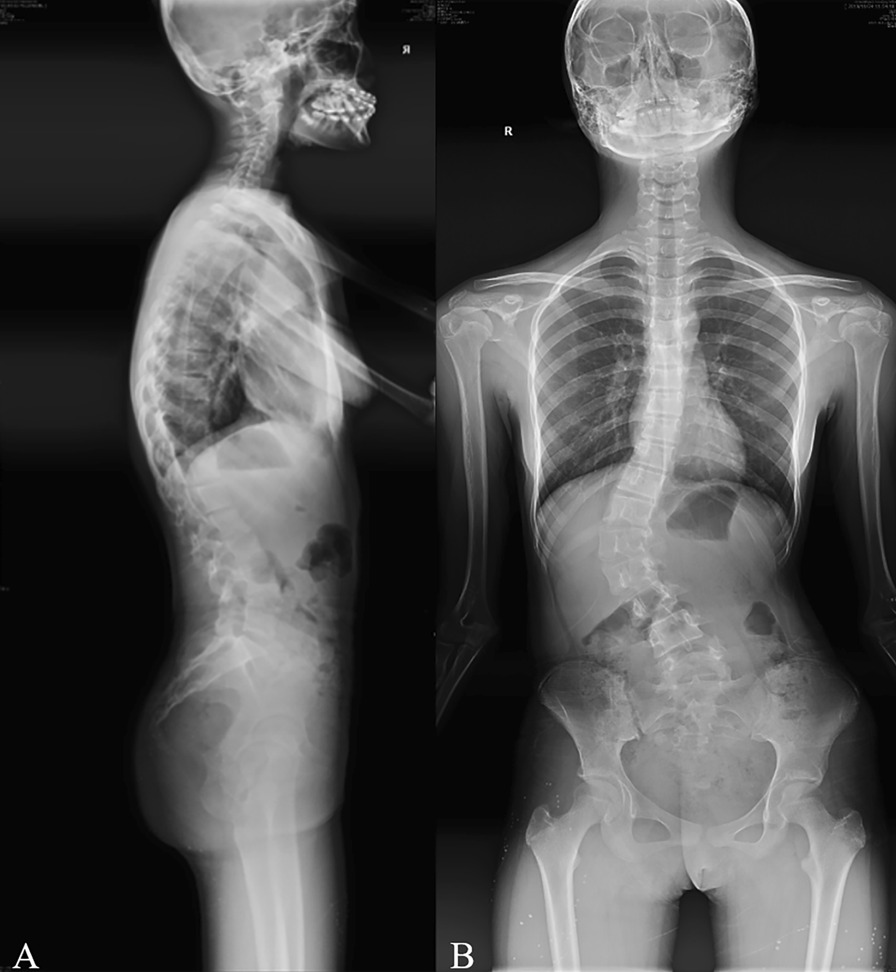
Biplane X-ray of the patient with Lenke5 AIS. A Lateral film. B Frontal film
